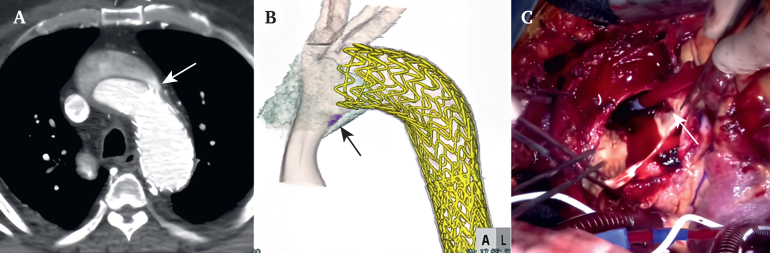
Pre-operative examination and operative findings in retrograde type A aortic dissection cases. (A,B) Pre-operative computed tomography shows an intimal tear (arrow) adjacent to the proximal end of the stent graft. (C) Intra-operative findings also show an intimal tear on the lesser curvature of the arch close to the proximal end of the stent graft (arrow).
